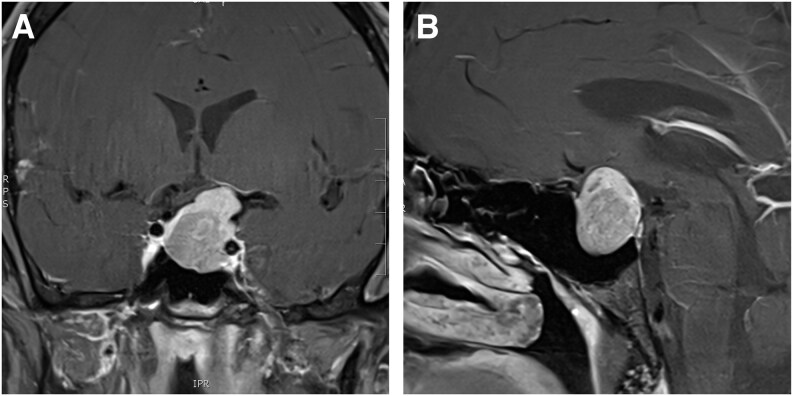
Magnetic resonance imaging (A) coronal (B) sagittal of the brain showing a 24.7 × 18.8 × 26.7-mm macroadenoma deviating the left optic nerve and chiasm.
